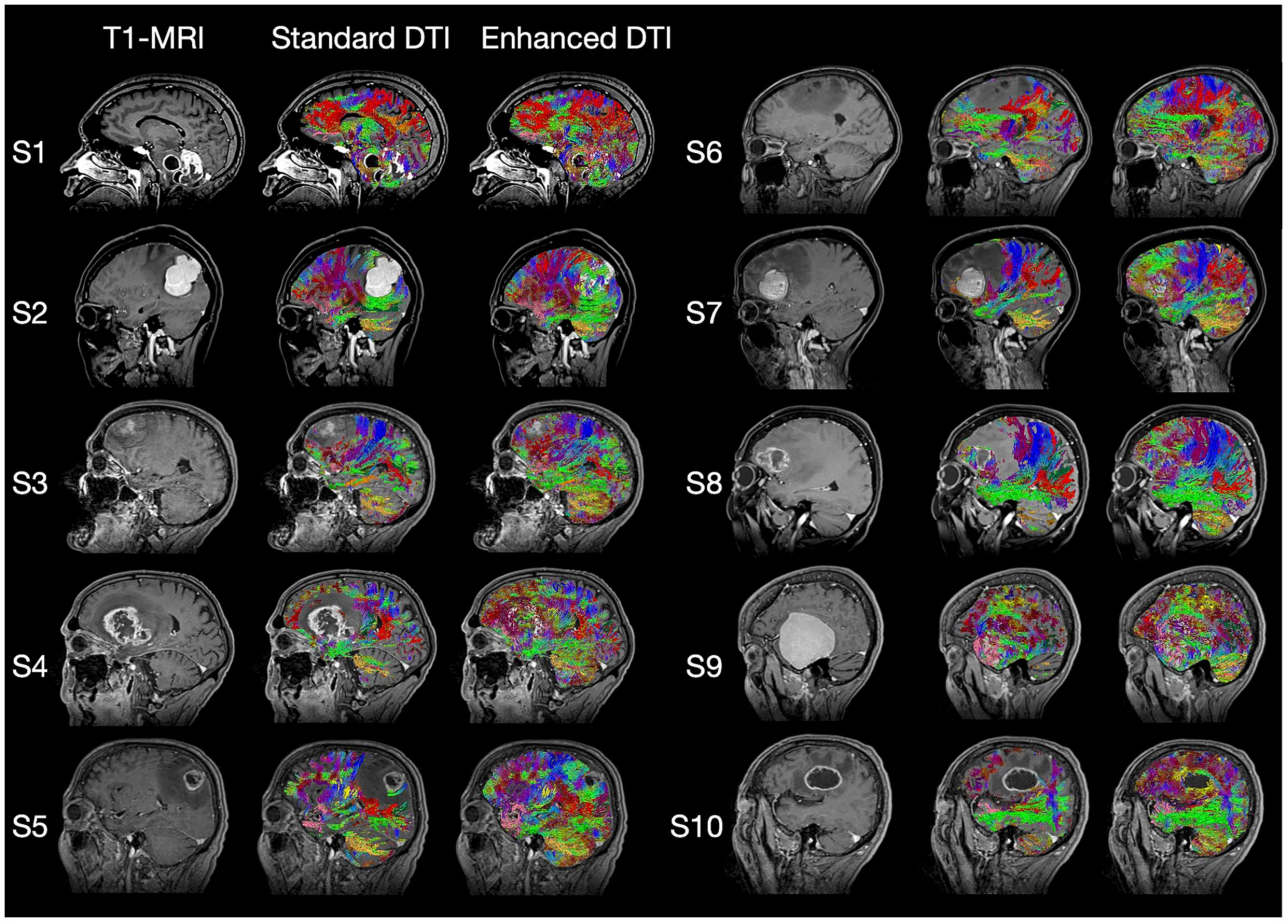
Standard T1-weighted MRI, Standard DTI Tractography, and Enhanced DTI Tractography neuroimages for each of the 10 subjects (i.e., patients) evaluated. Tract recovery profiles with enhanced DTI (Columns 3 and 6) will vary depending on the associated intra- and peritumoral dynamics of the underlying pathology, with each tumor type featuring distinctly varying degrees of tract invasion, mass effect, necrosis, and peritumoral edema impacting the recovery process.
